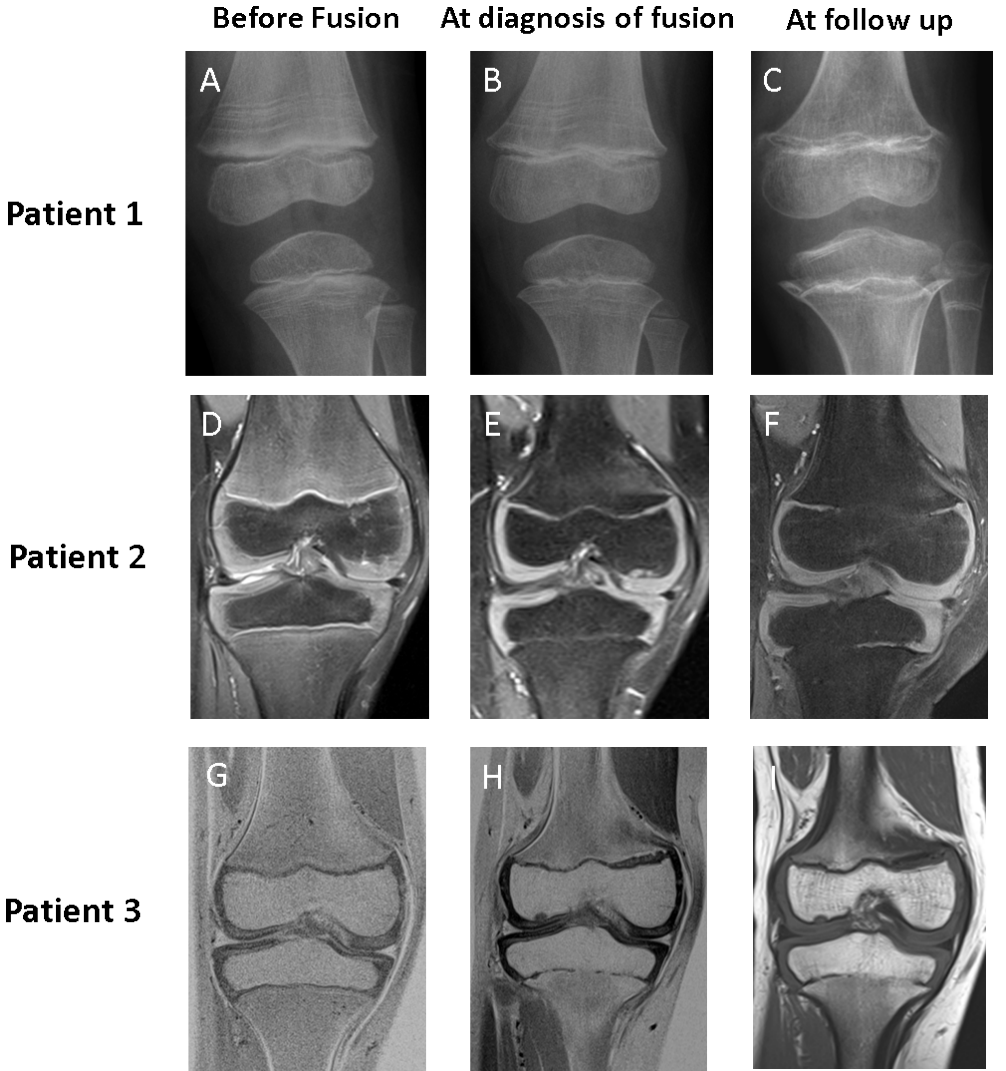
Imaging of knee in patients 1, 2, and 3 before fusion, at diagnosis of fusion, and at follow up Baseline imaging (Panels A, D, and G) show patent physes in all patients at the start of vismodegib therapy. In patient 1, physeal fusions were initially more pronounced in the proximal tibia, incomplete in the distal femur, and absent in the proximal fibula (Panel B). Follow-up radiography 17 months after stopping therapy (Panel C) showed progression of fusions in all physes and development of abnormal metaphyseal sclerosis. In patients 2 and 3, MRI showed that the initial identification of fusion was subtle, with the development of small bridging fusions at the completion of 12 cycles of vismodegib therapy (Panels E and H). Over time, these bridges widened to occupy more of the physeal stripe, as revealed by the MRI taken 6 months after the completion of vismodegib therapy (Panels F and I).
